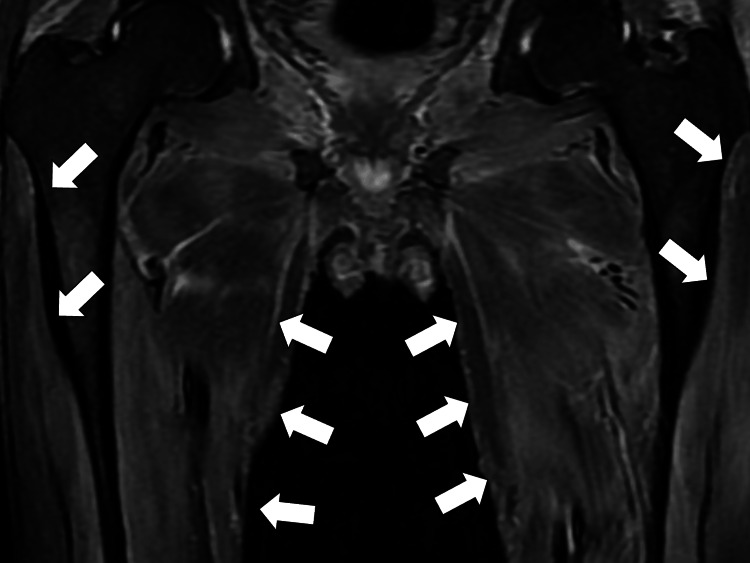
Magnetic resonance imaging (MRI) of both thighs showing high-signal areas in the muscles on short tau inversion recovery (STIR) imaging (white arrows)
